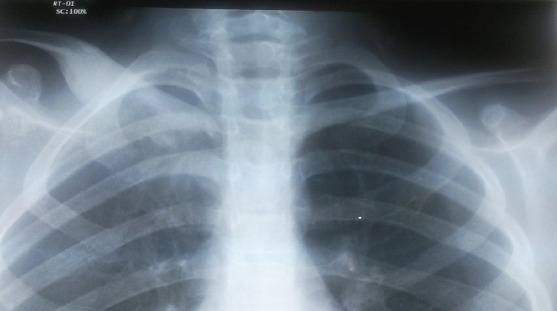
Radiographie standard des clavicules, aspect de condensation osseuse de la clavicule droite avec augmentation de son diamètre, sans lyse osseuse
